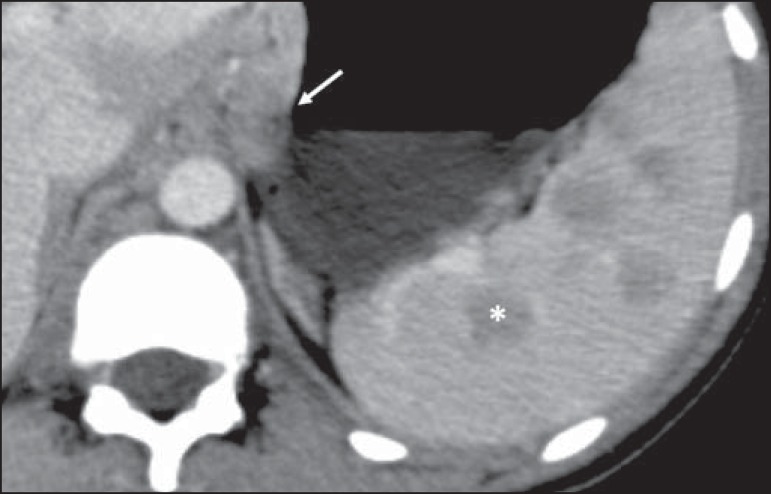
Multinodular splenic involvement by lymphoma. Contrast-enhanced axial CT
